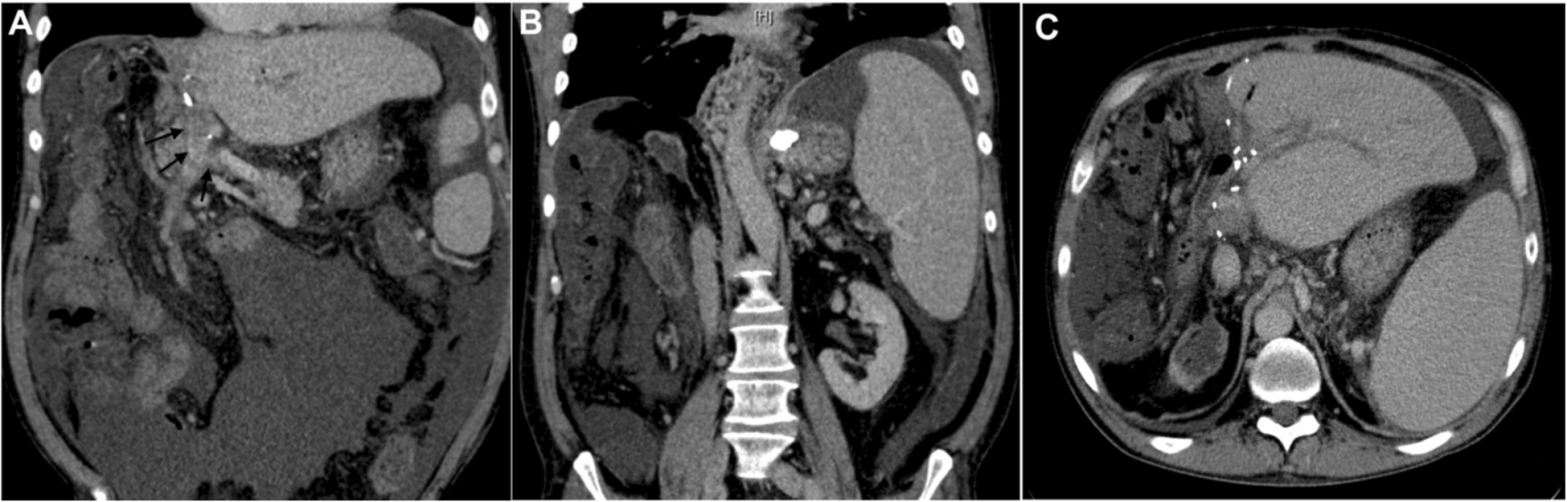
Pre-interventional Contrast-enhanced CT of the abdomen: A, B, C Coronal (arrows are pointing to the occluded portal vein) and axial venous phase images demonstrate the postoperative situs after hemihepatectomy, the cavernous portal vein occlusion with extension to the confluence, ascites and splenomegaly.
